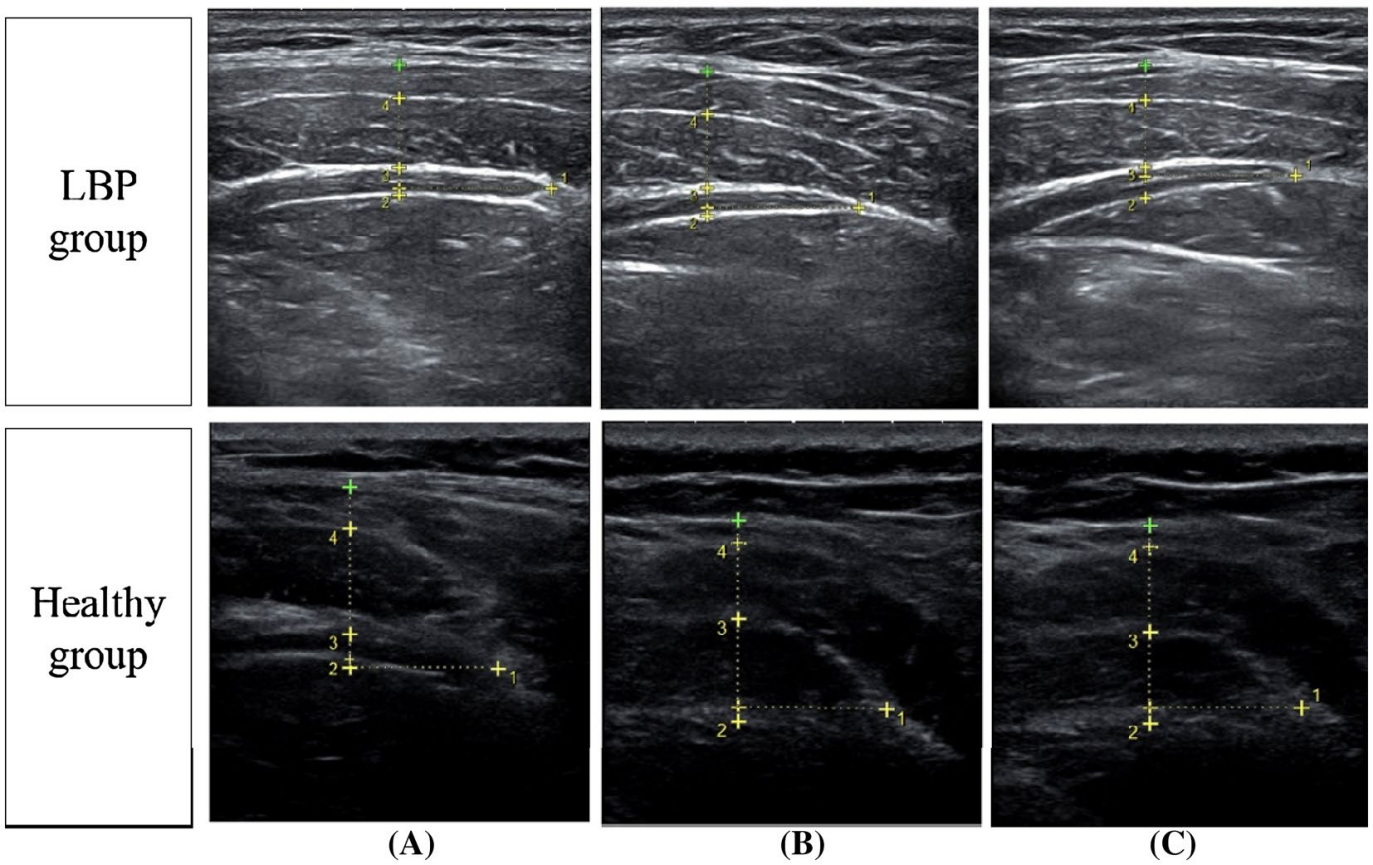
Measurement of the thickness of the transverse abdominis, external oblique, and internal oblique muscles using ultrasonography in the (A) supine, (B) usual sitting, and (C) upright sitting positions, in lower back pain (LBP) group and healthy controls.
